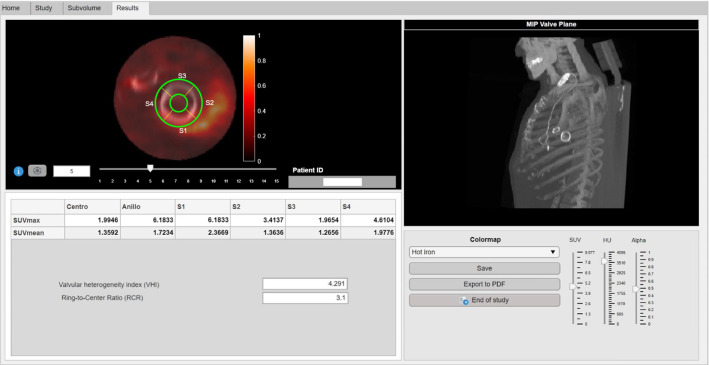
Results module*. The exploration of the performed segmentation to adequately interpret the obtained results is aimed at this module. The fusion image of the subvolume encompassing the heart valve is shown. To anatomically localize the valve-segments, a maximum intensity projection image of the valve plane is shown. Quantitative SUV image features regarding to the segmentation regions as well as heterogeneity defined ratios are provided. Data exporting options are also permitted
